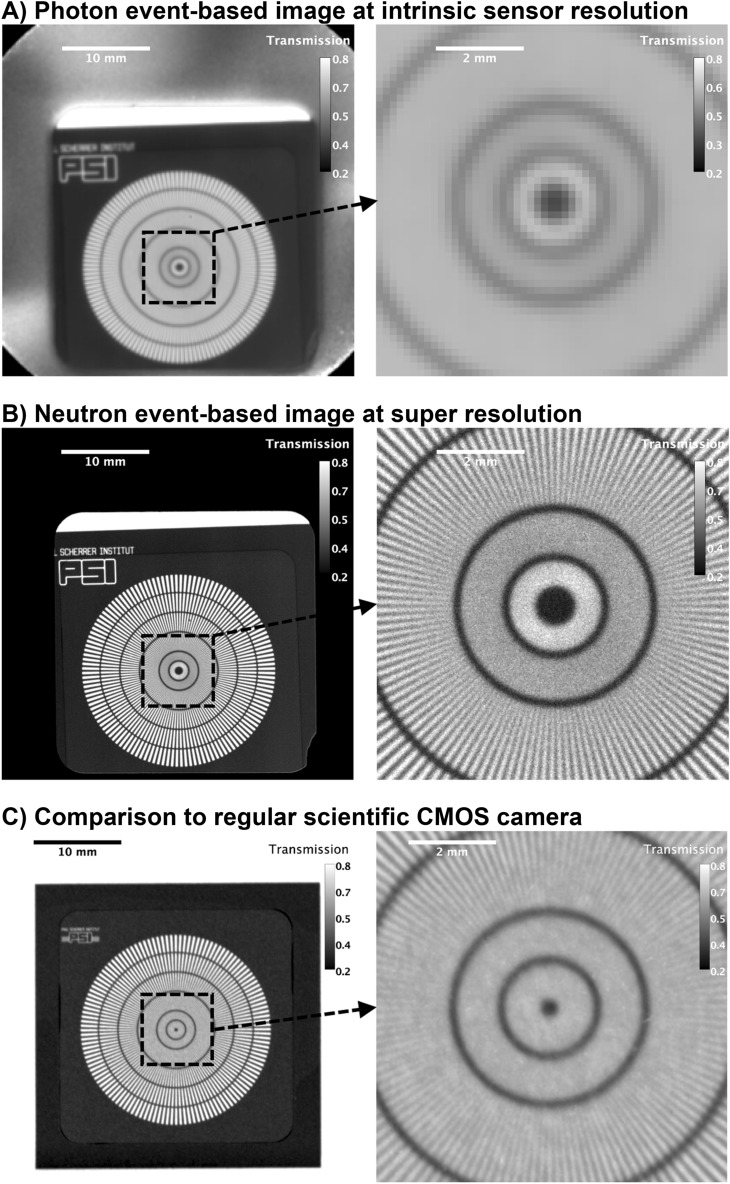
Neutron radiographs of resolution pattern. Photon event-based image recorded with TPX3Cam at intrinsic sensor resolution using 125 μm ^6^LiF:ZnS scintillator in (A). Neutron CoM event-based image recorded with TPX3Cam at sub-pixel sensor resolution of 0.1 × pixel-pitch using 125 μm ^6^LiF:ZnS scintillator and TPX3Cam in (B). For comparison, regular neutron radiograph recorded with scientific CMOS camera (ANDOR NEO) using 50 μm ^6^LiF:ZnS scintillator in (C).
